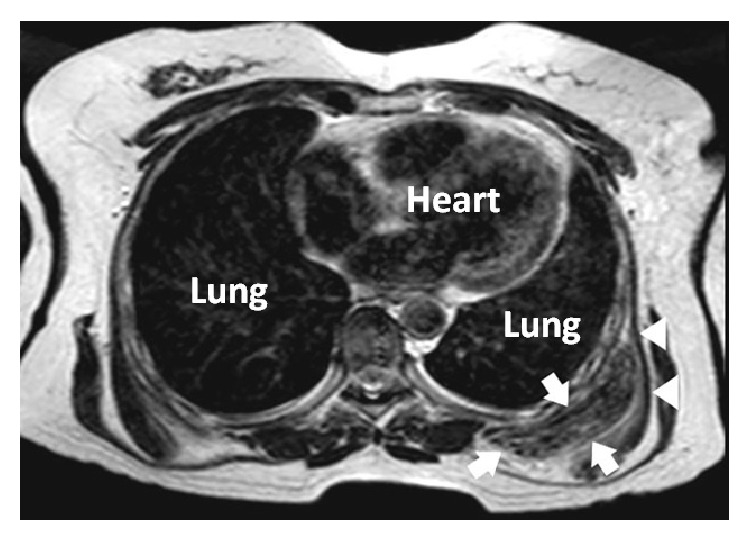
The T1 and T2 weighted MRI examination of the mass showed a localized heterogeneous mass of 64 × 16 mm adjacent to the left scapula. Please note the hyperintense adipose tissue in the form of lines and hypointense fibrous tissue.
